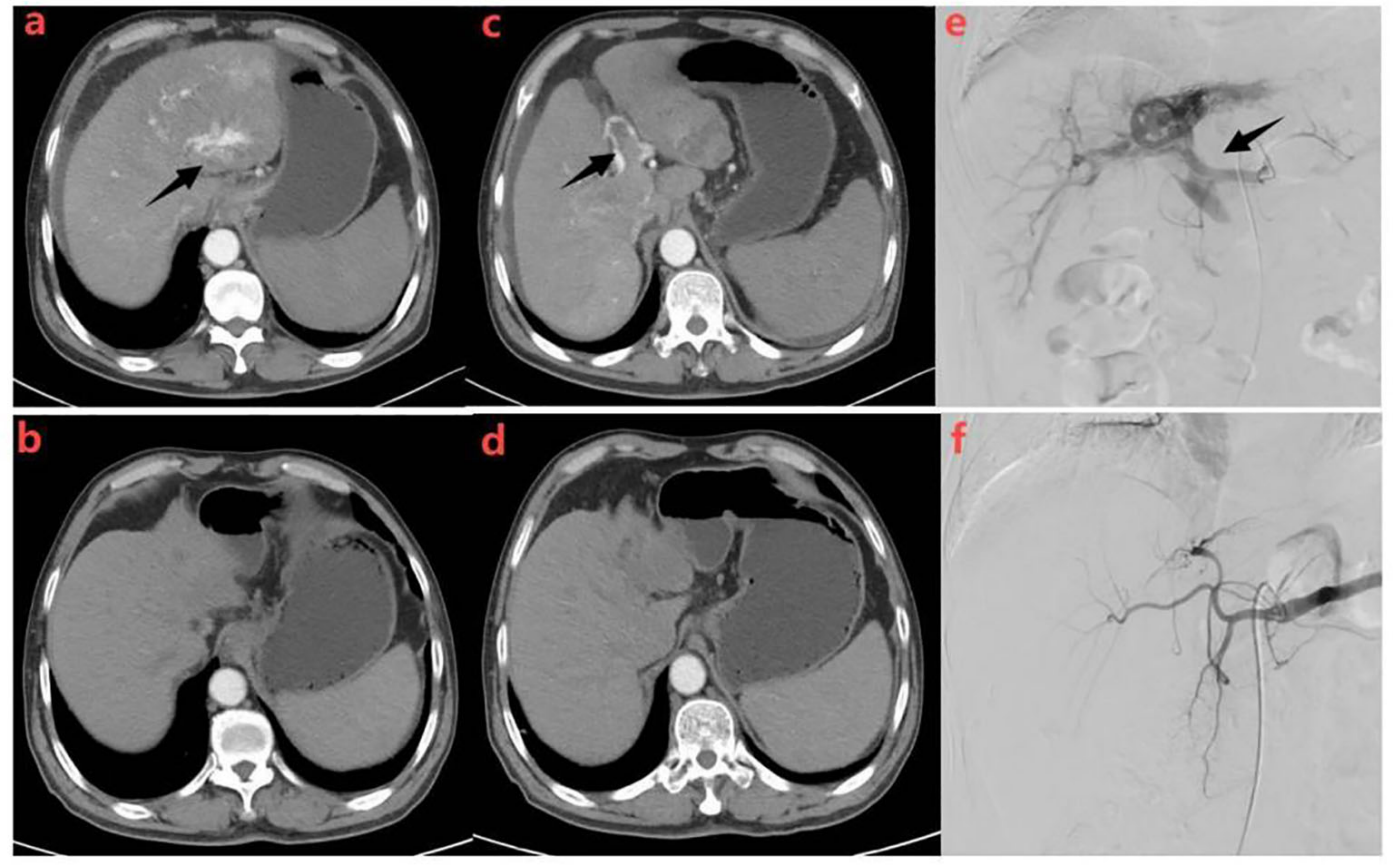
Representative radiographical images of a patient who achieved APS disappearance after treatment. Images of man aged 58 years with HCC complicated by Vp4 PVTT and severe APS after receiving triple therapy.Axial contrast-enhanced CT images in the arterial phase (a) and portal phase (c) before the initiation of treatment. CT images taken during the arterial phase (a) displayed early enhancement of portal vein branches (indicated by a black arrow), representing APS. Portal venous phase (c) showed filling defects in the main trunk and left branch of the portal vein, representing tumor thrombi in the portal vein (indicated by a black arrow).Hepatic angiography (e) revealed severe APS and Vp4 PVTT(indicated by a black arrow). Subsequent axial contrast-enhanced CT images in the arterial (b) and portal (d) phase were taken after three cycles of triple therapy, showing no apparent APS and regression of portal vein tumor thrombus. Hepatic angiography in image (f) confirmed the absence of APS.
